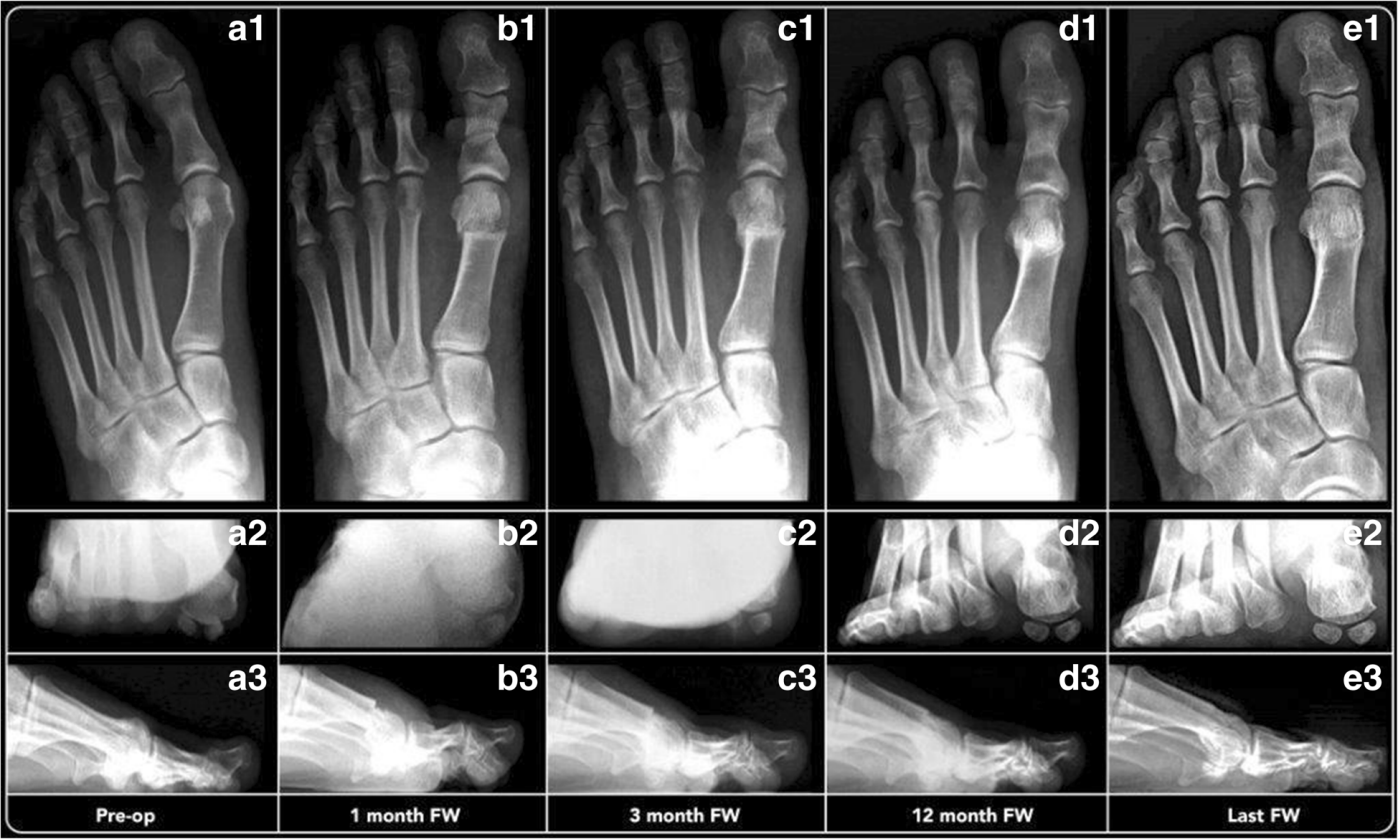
A 36-year-old woman with mild HV: *1* antero-posterior radiographic images, *2* sesamoid and *3* lateral view at preoperative period (*a*), 1-month follow-up (*b*), 3-month follow-up (*c*), 12-month follow-up (*d*) and 48-month follow-up (*e*), showing the maintained correction of the deformity
